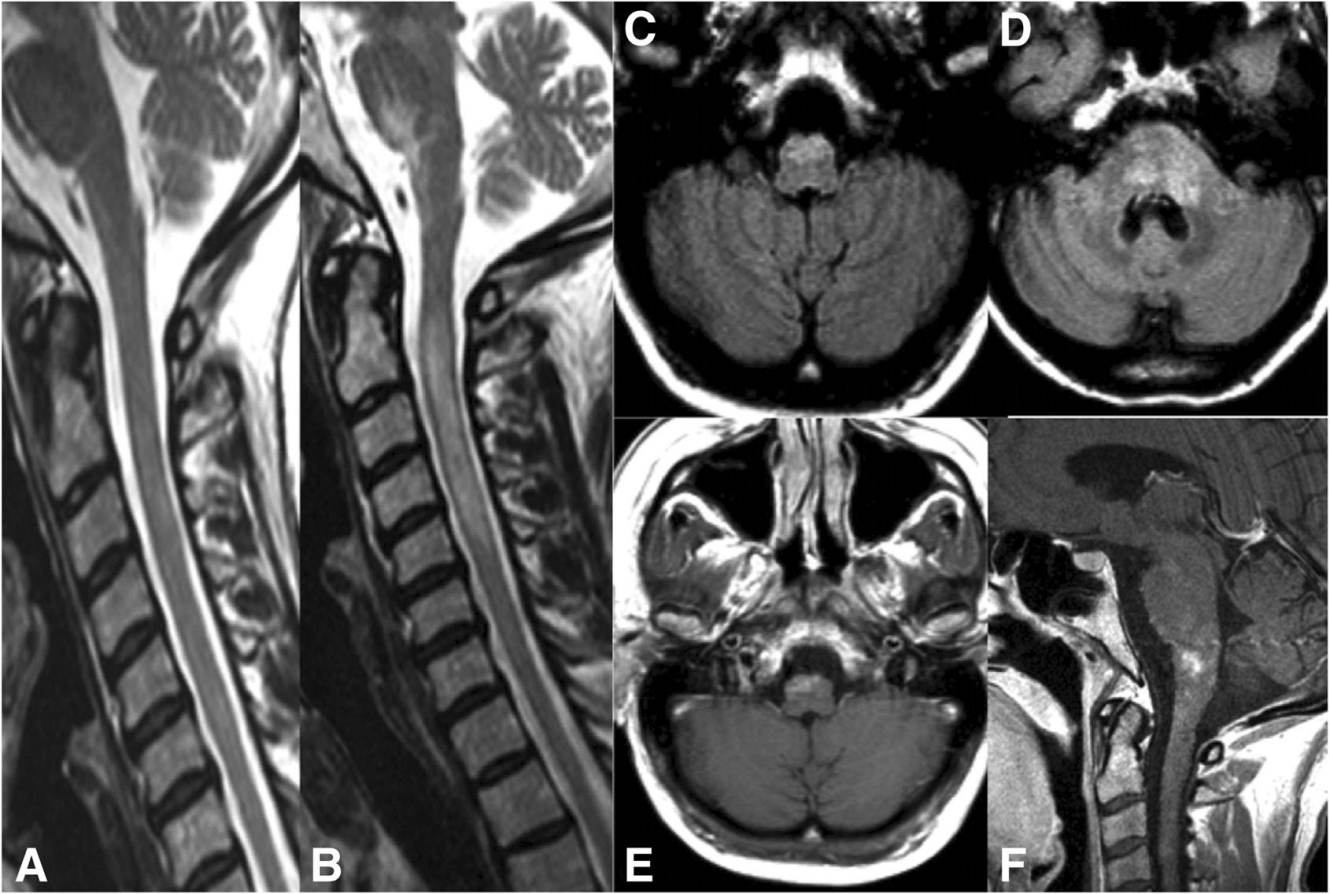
Serial MRI examination of patient 1. a Sagittal T2 weighted baseline MRI showing no involvement of the brainstem or of the spinal cord. b-e Follow-up MRI 5 month later revealed a new spinal cord lesion extending over 4 vertebral segments with cord swelling on T2-weighted imaging as well as new lesion formation in the pons and medulla oblongata with gadolinium enhancement on T1-weighted imaging. Within the following 5 months clinical symptoms deteriorated further with infratentorial T2 lesion enlargement and new lesion formation in the pons, e.g. adjacent to the middle cerebellar peduncle, accompanied by gadolinium enhancement (f)
